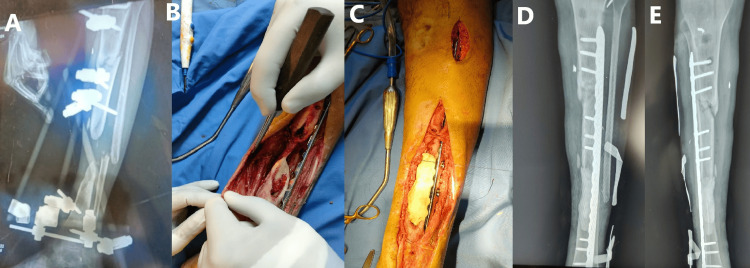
(A) Preoperative leg X-ray showing a segmental tibial fracture with bone loss. An ankle-spanning external fixator is visible, with three pins on the proximal tibia, one on the distal tibia, and a transcalcaneal pin. (B) Intraoperative image displaying significant bone loss, with the posterior tibial nerve visible in the field. (C) Intraoperative image showing fracture fixation using an LCP plate and bone cement to fill the gap as part of the first stage of the Masquelet technique. The plate was inserted percutaneously to span the proximal fracture. A second stage is planned to replace the bone cement with bone graft after six weeks. (D, E) Anteroposterior and lateral leg X-rays showing tibial fracture fixation with bone cement filling the gap. The white shadow surrounding the leg is from radiopaque-marked gauze. LCP: locking compression plate
